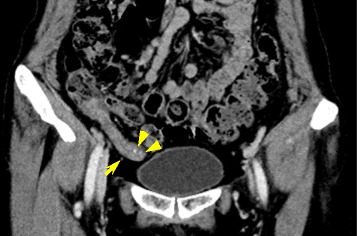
Contrast-enhanced abdominal computed tomography (CT). A thickened appendix, without signs of inflammation, including fat stranding and extra-luminal fluid, was observed in the coronal plane (*yellow arrow*). There were focal calcifications in the appendiceal wall (*yellow arrowheads*)
